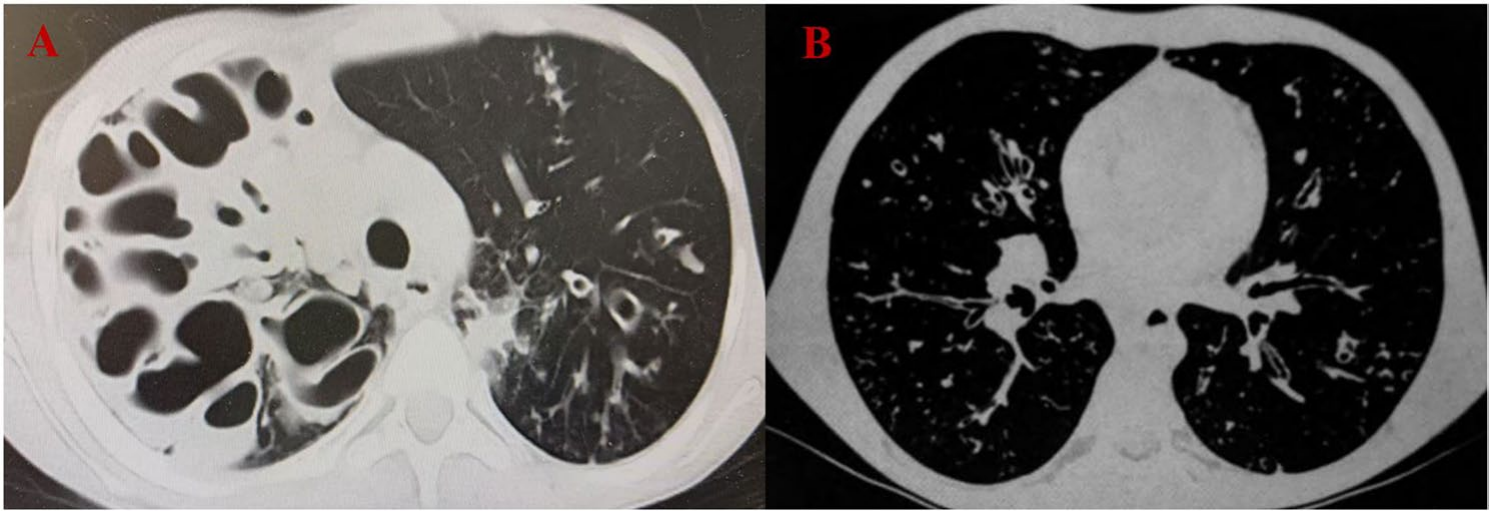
Chest CT images of children diagnosed with CF. (A) Chest CT of patient 8 shows extensive bronchial dilation within hyperdense lesions in the right lung; multiple bronchial wall thickening and luminal dilation are identified in the left lung, with some lesions showing the signet ring. (B) Chest CT of patient 1 showed multiple bronchial wall thickening and bronchial luminal dilation in both lungs, as well as small centrilobular nodules in the right lung
